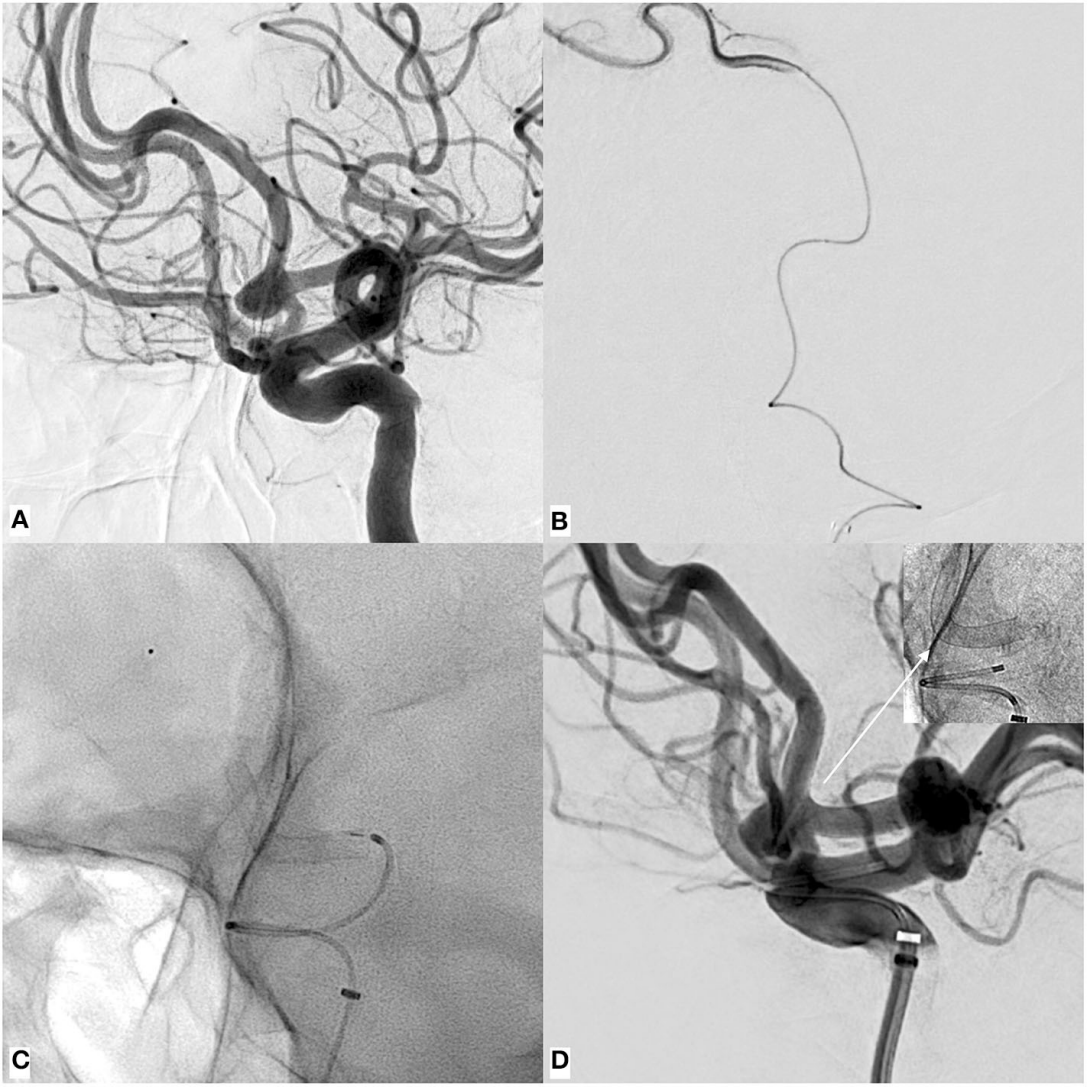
An example of p48MW hydrophilic coating (HPC) implantation for treatment of an un-ruptured, broad-based aneurysm of the AcomA complex *via* the left internal carotid artery (ICA). (A) Digital subtraction angiography (DSA) run in working projection revealing the broad based (neck: 5.3 mm), saccular aneurysm (fundus: 5.2 × 4.3 mm) predominantly filled by the left anterior cerebral artery (ACA). (B) Catheterization of the elongated, demanding ACA using the Excelsior SL 10 and confirmation of the correct endoluminal position distal to the aneurysm as a pre-requisite for safe microcatheter exchange/introduction of the Prowler Select Plus required for device delivery. (C) Plain radiography in working projection demonstrating flow diverter stent (FDS) implantation—note the radiopaque olive tip at the distal end of the p48 wire at the level of the distal A2 segment, which helped to stabilize the equipment during deployment. Implantation of the device has caused significant straightening of the A1–A2 transition, additionally contributing to the redirection of blood flow away from the aneurysmal orifice. (D) Control injection after implantation shows the patency of the vessel in combination with the location of the implanted device (radiogram upper right corner).
